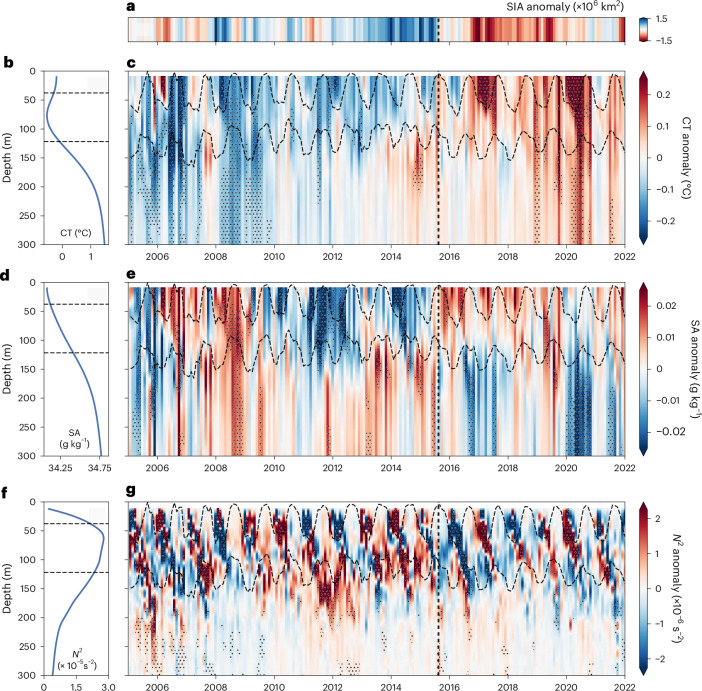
Vertical hydrographic anomalies. a, The monthly Southern Ocean SIA anomaly. b–g, The spatially weighted mean (b, d and f) and monthly anomaly profiles (c, e and g) of conservative temperature (CT; b and c), absolute salinity (SA; d and e) and Brunt-Väisälä frequencyBrunt-Väisälä frequency (stratification) (N^2^; f and g) in the seasonally ice-covered Southern Ocean. The vertical dashed black lines in a, c, e and g denote the start of the transition from high SIA to low SIA (August 2015). The stippling in c, e and g indicates regions where the anomaly is more than one standard deviation for each depth level. The dashed black horizontal lines in b–g denote the upper and lower WW boundaries.
